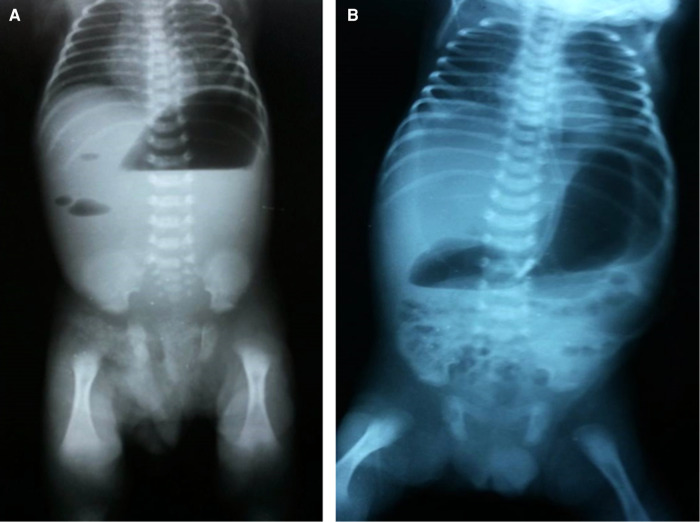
Erect x-ray of the abdomen. (A) At admission. (B) Three days postoperatively.
